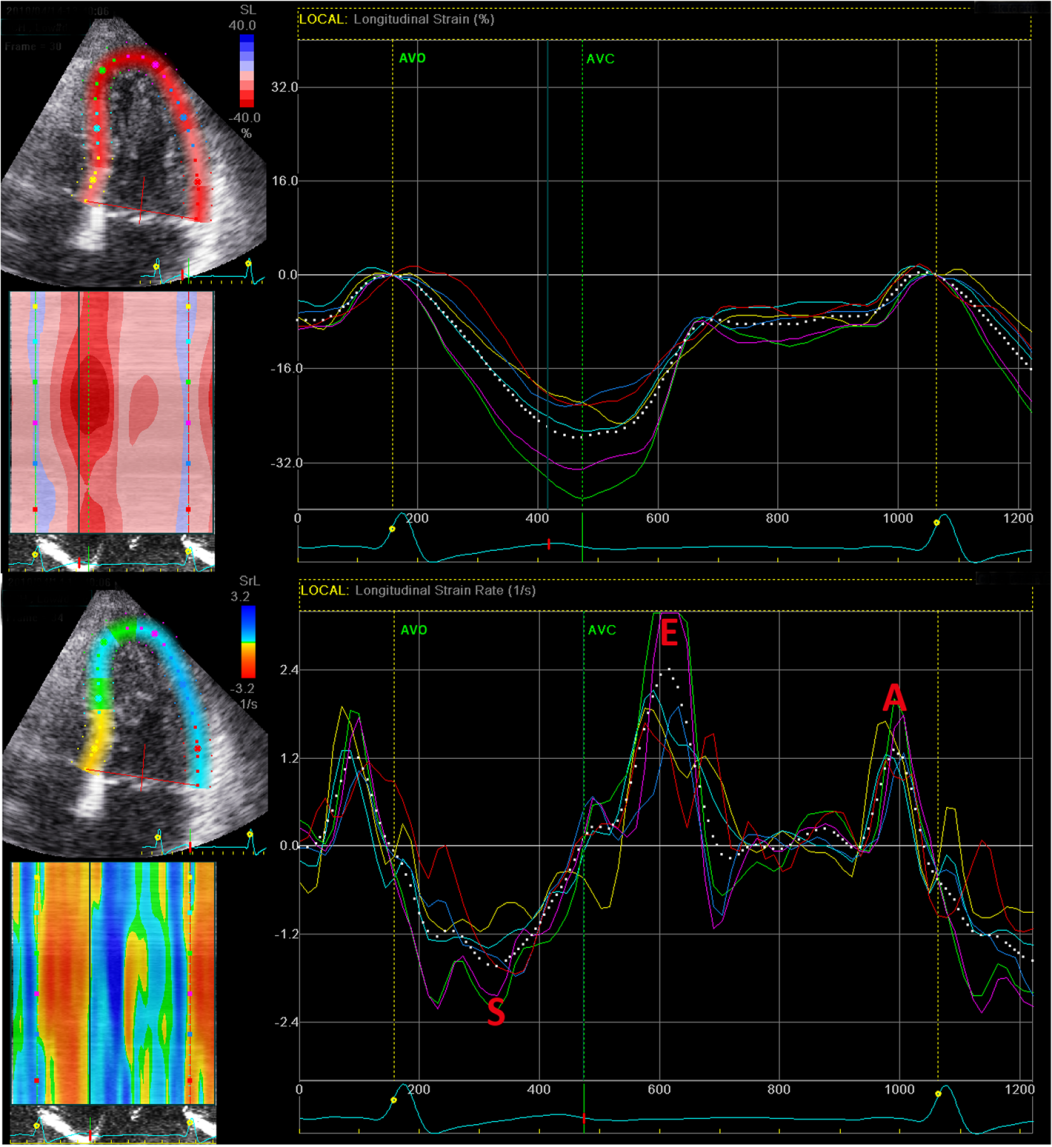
Longitudinal strain and strain rate imaging by 2D speckle tracking for the apical 4-chamber view. The top panel is the strain throughout one cardiac cycle for each of the color coded LV segments (mean strain shown by dotted white line). In this example, the mean peak strain is about 27% which occurs during LV ejection, defined as the interval between aortic valve opening (AVO) and closure (AVC). The bottom panel shows the corresponding LV strain rate. Note the negative strain rate in systole (S) and the positive strain rates (E during early diastole, and A during late diastole).
